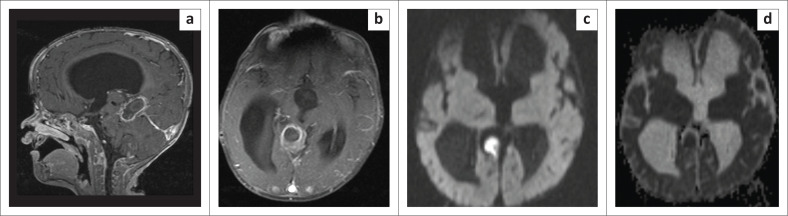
Follow-up MRI at 6 months post-procedure showed a resolving chemical abscess: (a) T1-fat-saturated post-contrast mid-sagittal, (b) T1-fat-saturated post-contrast axial, (c) Diffusion-weighted imaging axial at b 1000 s/mm^2^ and (d) Apparent diffusion coefficient axial at same level as 4c showing further reduction in the size of the remnant sac and hydrocephalus, persistent peripheral rim enhancement and restricted diffusion.
